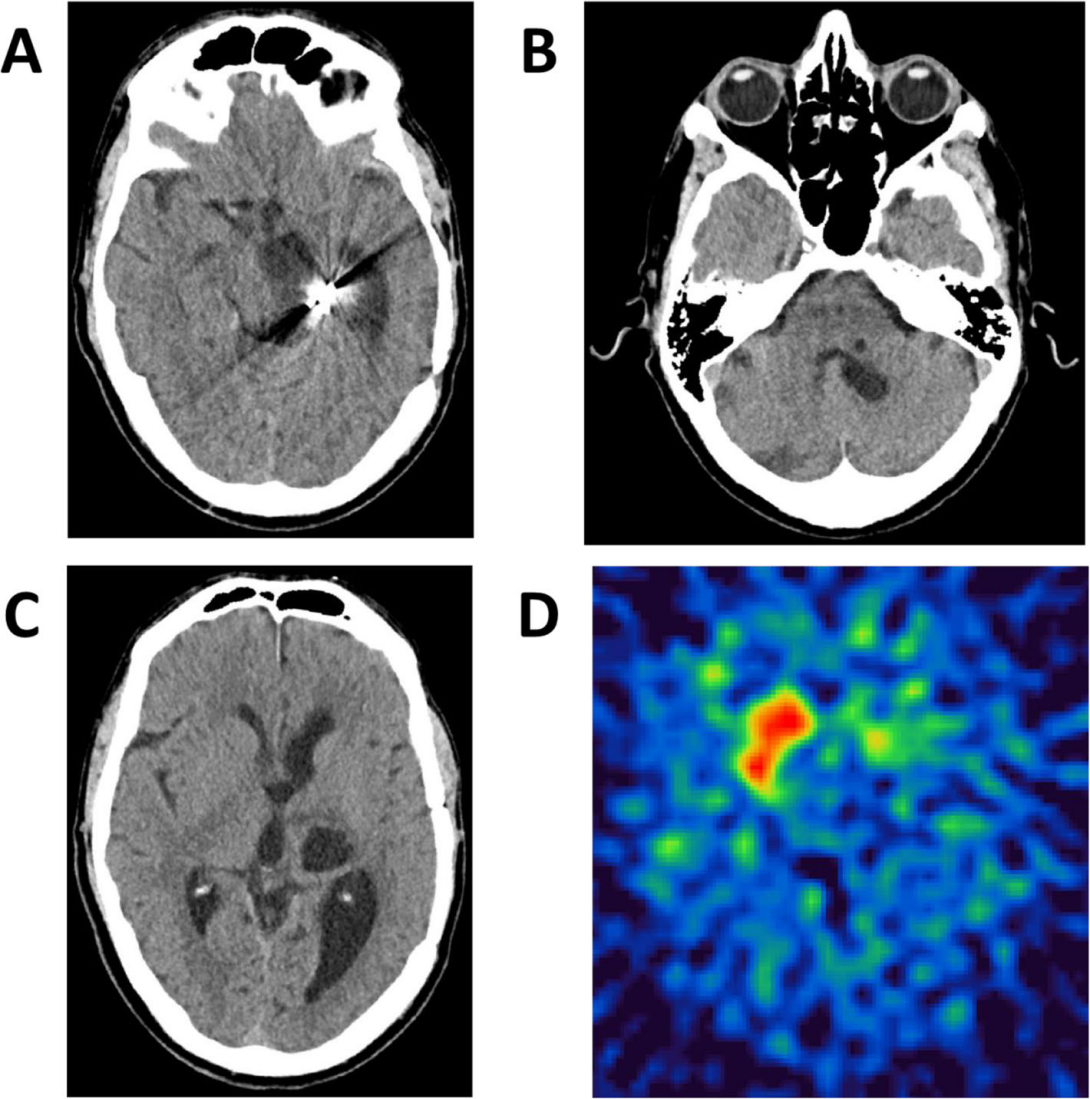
Arachnoid cysts in a patient suffering from secondary right-sided hemiparkinsonism with superimposed hemiataxia and spastic hemiparesis. CCT-scans showing (a) the aneurysma clip, (b) pons, (c) cystic lesions and ventricular enlargement, as well as (d) DAT-SPECT imaging showing severe striatal dopaminergic denervation on the left side. Also note the artefacts for the previously clipped aneurysm
